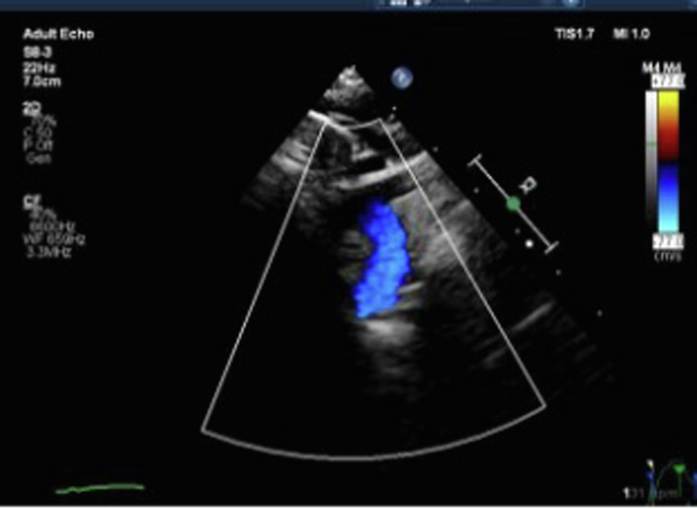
Follow-Up Transthoracic Echocardiography, Suprasternal View, Demonstrating the Aortic Arch 1 Month After Surgical Repair (Patient 1)
